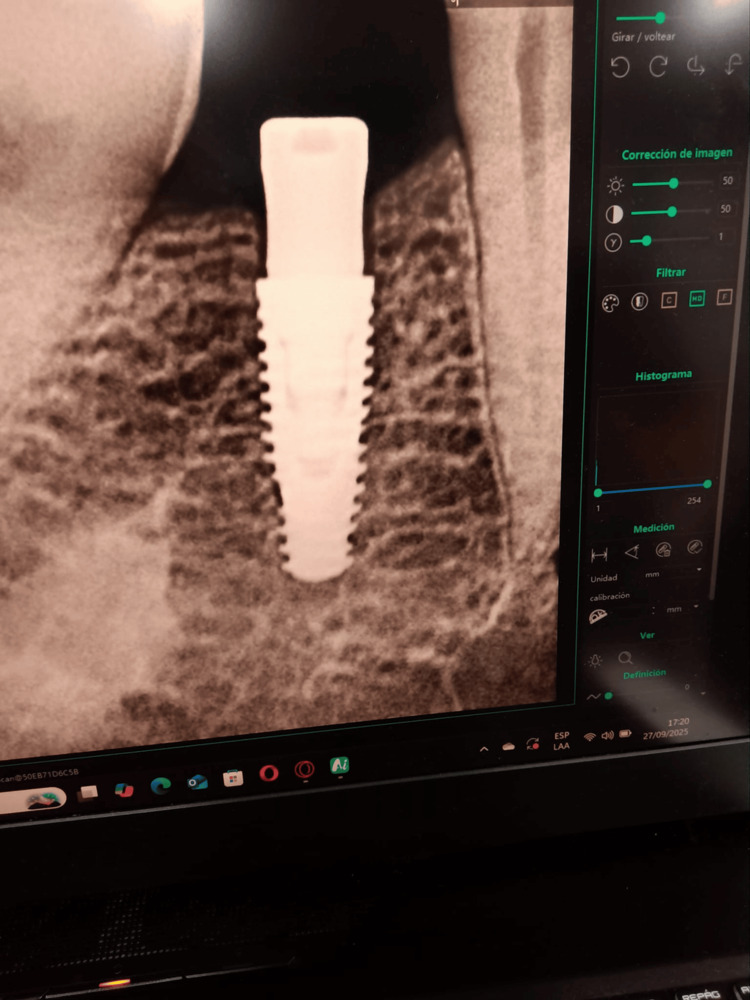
Postoperative radiograph showing implant placement at tooth #30. Periapical radiograph of the mandibular right first molar site (Universal #30; FDI 46) after implant placement. The implant fixture is visible within the alveolar bone, with adjacent teeth and surrounding bone structures observable.
